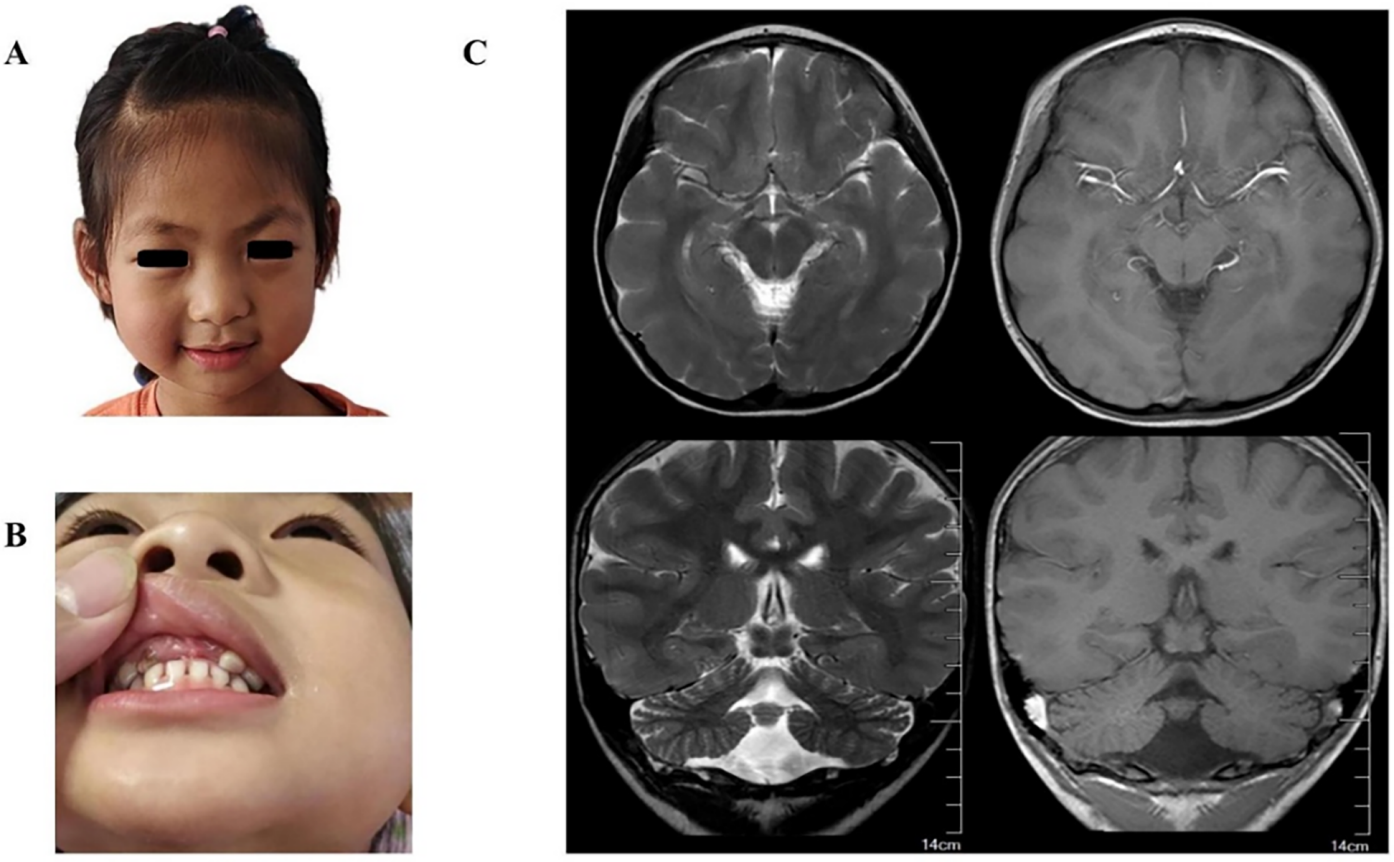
(A) photograph of the patient at 6 years showed narrow forehead, thick eyebrows, bulbous nose, smooth philtrum. (B) Photograph of the patient showed widened and separated teeth. (C) Brain MR images showed temporal, parietal and occipital pachygyria (the right is more prominent).
